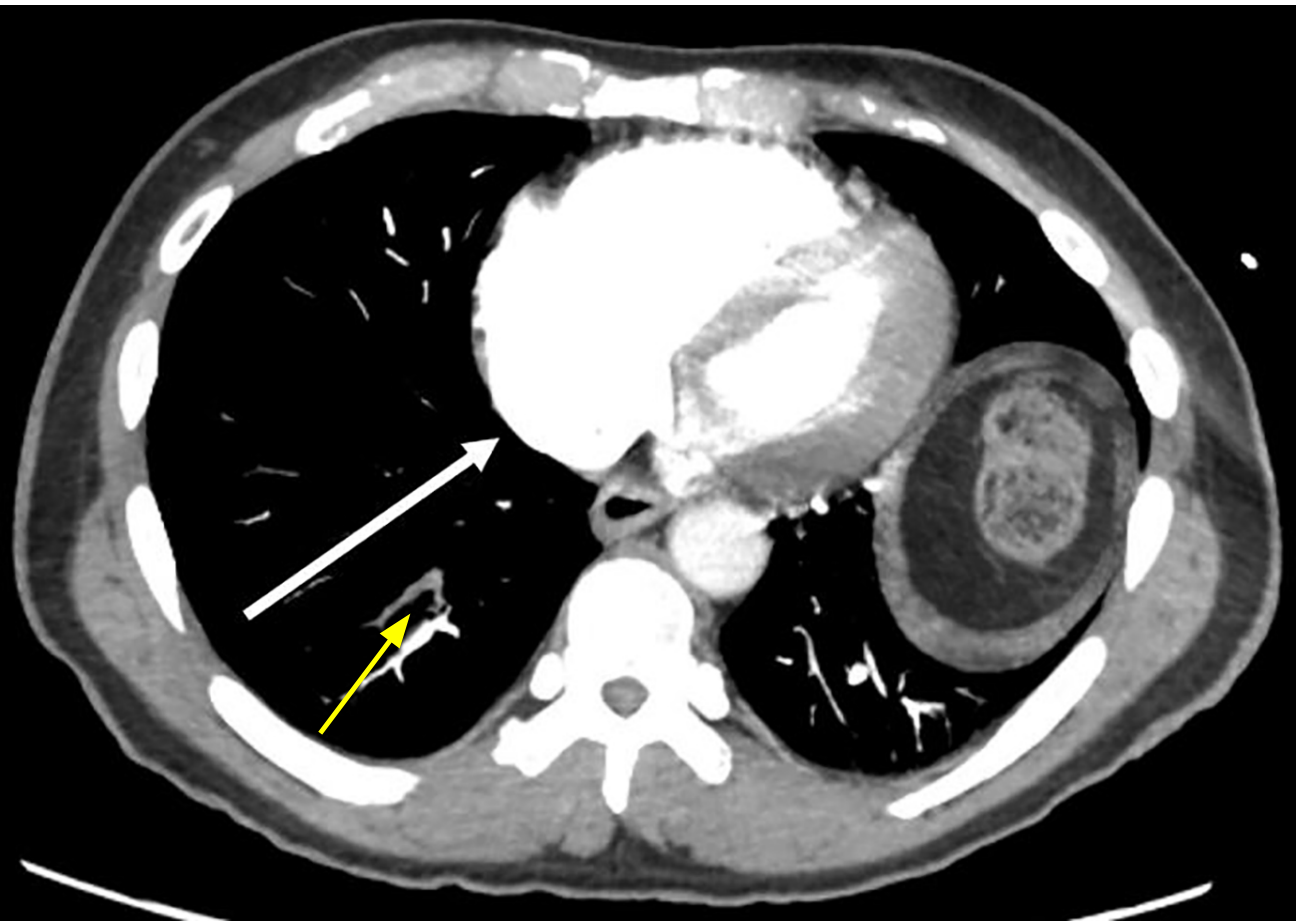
Axial image from computed tomography angiogram of the chest demonstrating significantly dilated right ventricle (arrow) and filling defect consistent with pulmonary embolism (yellow arrow).
